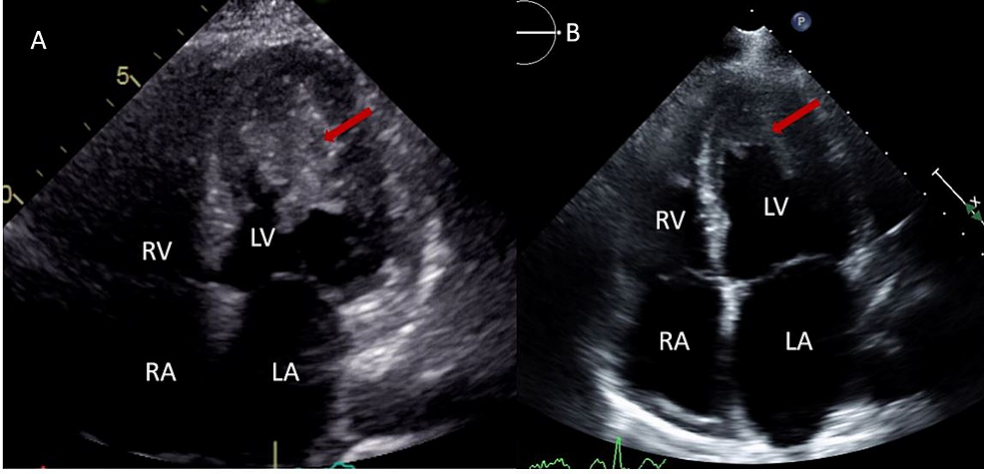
A TTE revealing a gigantic LV apical mass prior to anti-coagulation (left). A repeat TTE revealing significant resolution of LV thrombus after three months of anti-coagulation (right). TTE, transthoracic echocardiogram; LV, left ventricle; RV, right ventricle; LA, left atrium; RA, right atrium
